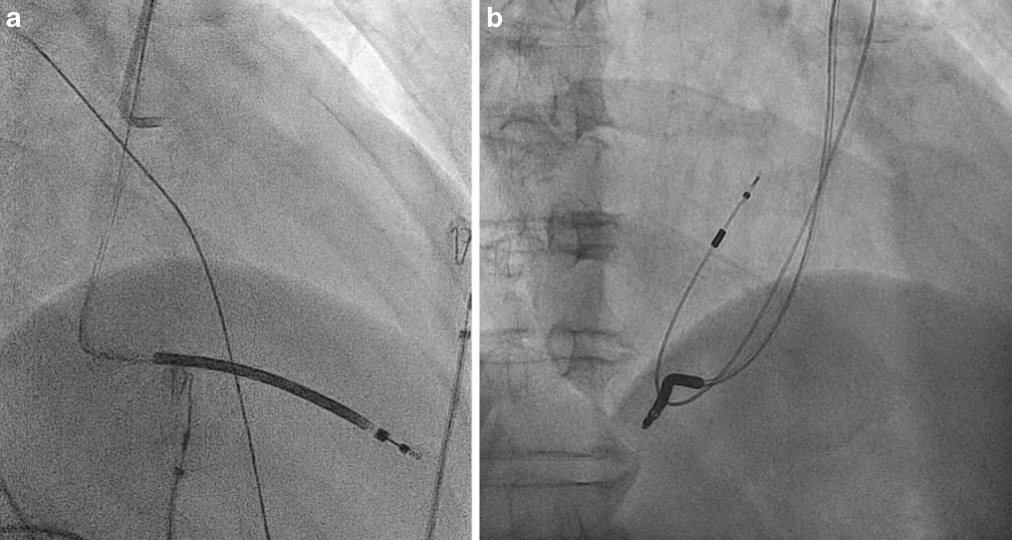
X-ray right anterior oblique view showing an apical lead position at implant (*left panel*) and displacement into the right atrium in the post-shock X‑ray (*right panel*)
